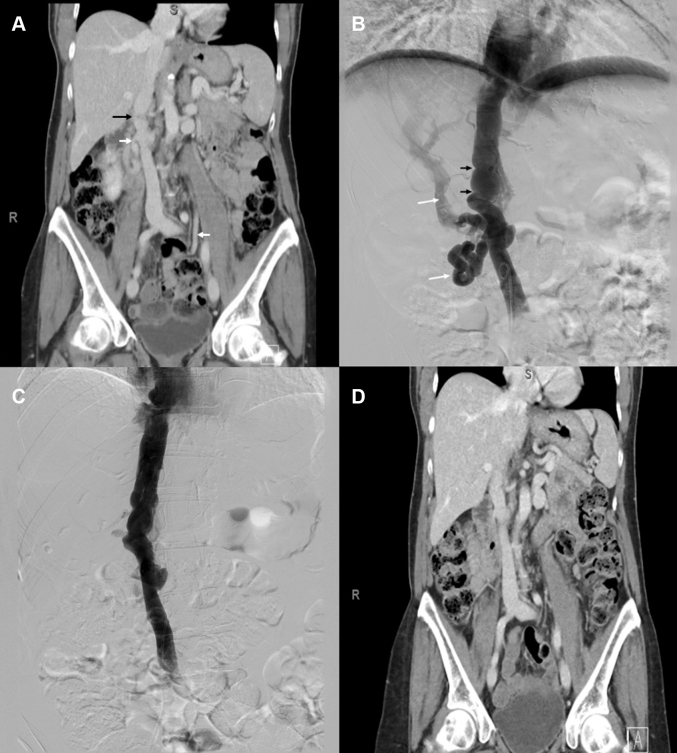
Computed Tomography Venography and Catheter Venography (A) Coronal view of the preprocedure computed tomography (CT) venogram of the abdomen and pelvis shows high-grade stenosis of the infrahepatic inferior vena cava (IVC) (black arrow) with numerous venous collaterals (white arrow). (B) Invasive venography before percutaneous intervention demonstrates multifocal IVC stenosis (black arrow) with severe collateral development (white arrows) in the infrahepatic IVC. (C) Venogram performed immediately after balloon angioplasty shows disappearance of venous collaterals with improved flow through the IVC. (D) Coronal view of CT venogram during follow-up shows continued resolution of collaterals.
